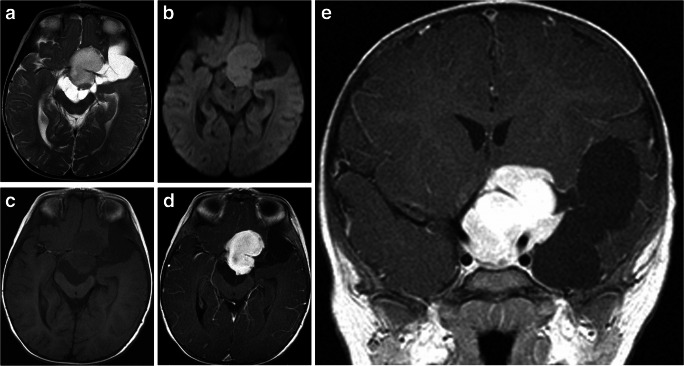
Pilomyxoid astrocytoma. a Axial T2-weighted sequence through the suprasellar region, demonstrating a large solid cystic tumor in the suprasellar region. The solid component is hyperintense compared to the gray matter and causes mass effects to the left frontal lobe. The amorphous cystic component conforms to the basilar cisterns, with splaying of the cerebral peduncles. b Axial diffusion image through the same level does not show diffusion restriction (ADC map not shown). c An axial pre-contrast T1-weighted sequence through the same level demonstrates that even the solid component of the tumor is hypointense compared to the adjacent gray matter. An axial post-contrast T1-weighted sequence through the same level (d) and the coronal T1-weighted sequence through the mid-sellar region (e) demonstrate intense enhancement of the solid component of the tumor. There is invasion of the left hypothalamus. The major arteries of the anterior circulation are surrounded by tumor on all sides. The cystic component extends into the left Sylvian fissure
